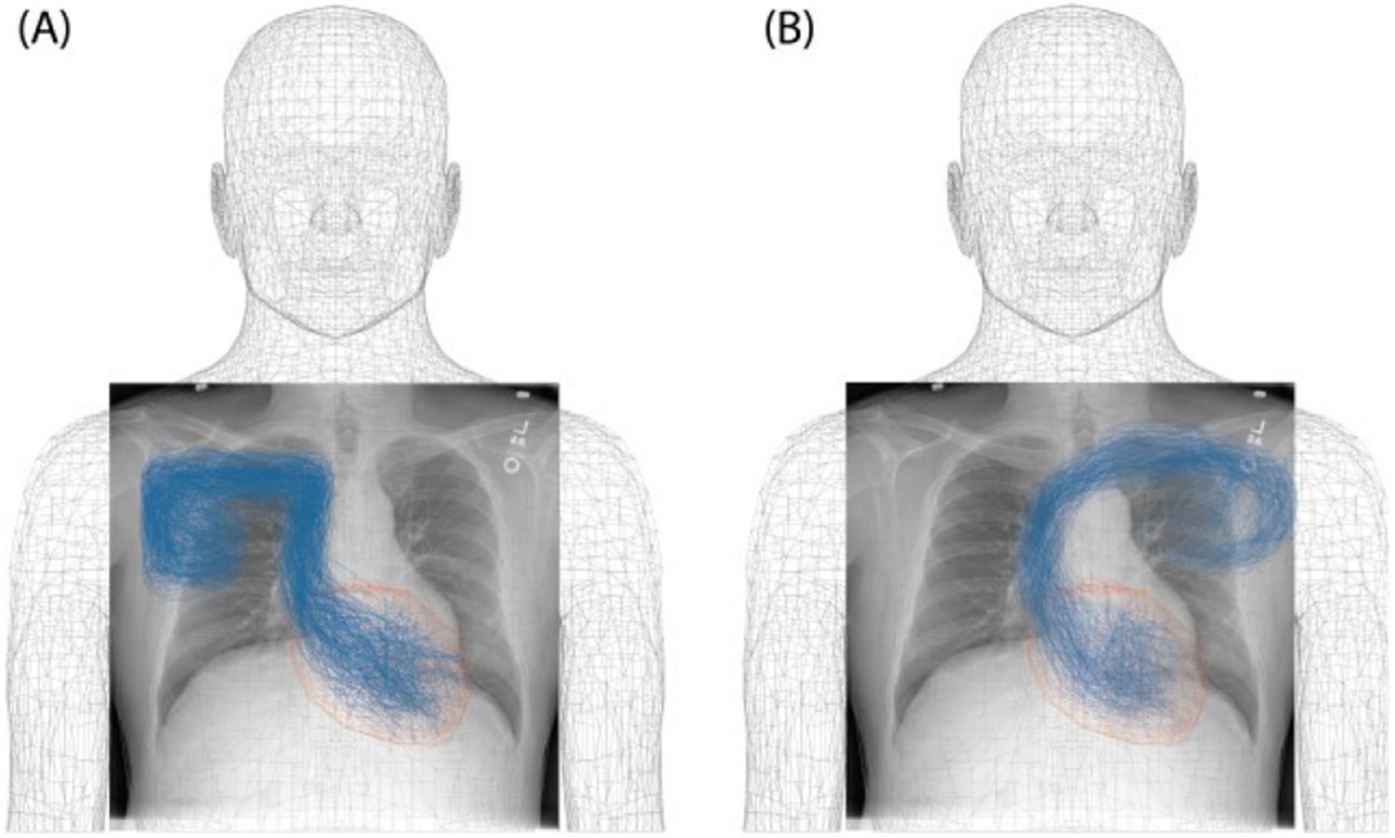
Example of X-ray photograph of a patient with CIED overlaid on ANSYS human body model and manual trajectories with IPGs on right (A) as well as left (B).
